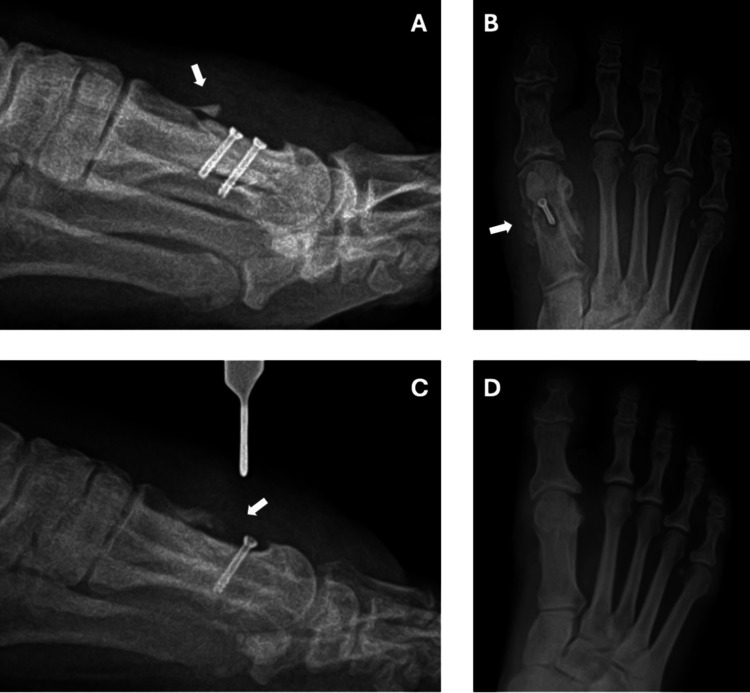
Radiographs of the patient’s right foot. A: four-week postoperative radiograph showing a small osseous fragment adjacent to the osteotomy site (arrow); B, C: radiographs obtained after revision surgery demonstrating persistent radiographic abnormalities, including focal bone erosion adjacent to the screw tract (arrows); D: four-year follow-up radiograph demonstrating complete osseous consolidation and resolution of prior abnormalities.
